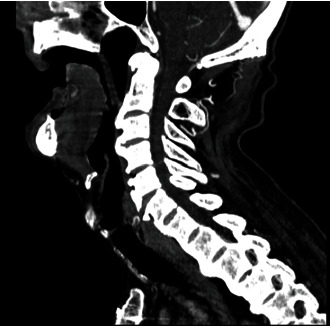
The sagittal CT scan of the patient's neck.
